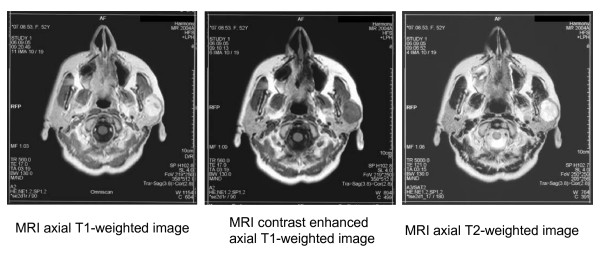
Pleomorphic Adenoma of the left parotid gland: MRI axial T1-weighted image, MRI contrast enhanced axial T1-weighted image and MRI axial T2-weighted image
